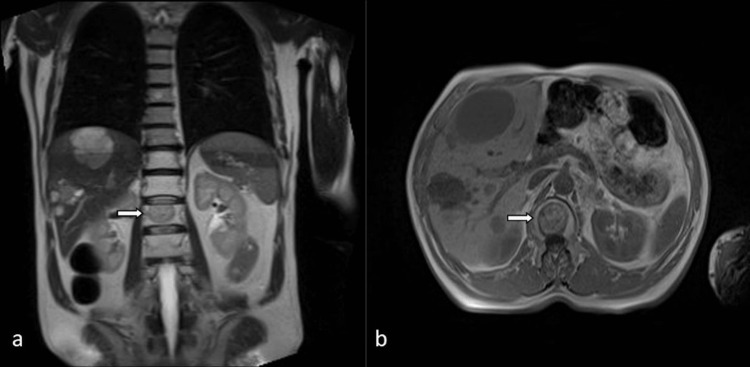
Vertebral metastases in a 61-year-old male patient. MR localizer sequence (a) and axial T1-weighted image (b) show sharply demarcated metastatic lesion (arrows).
